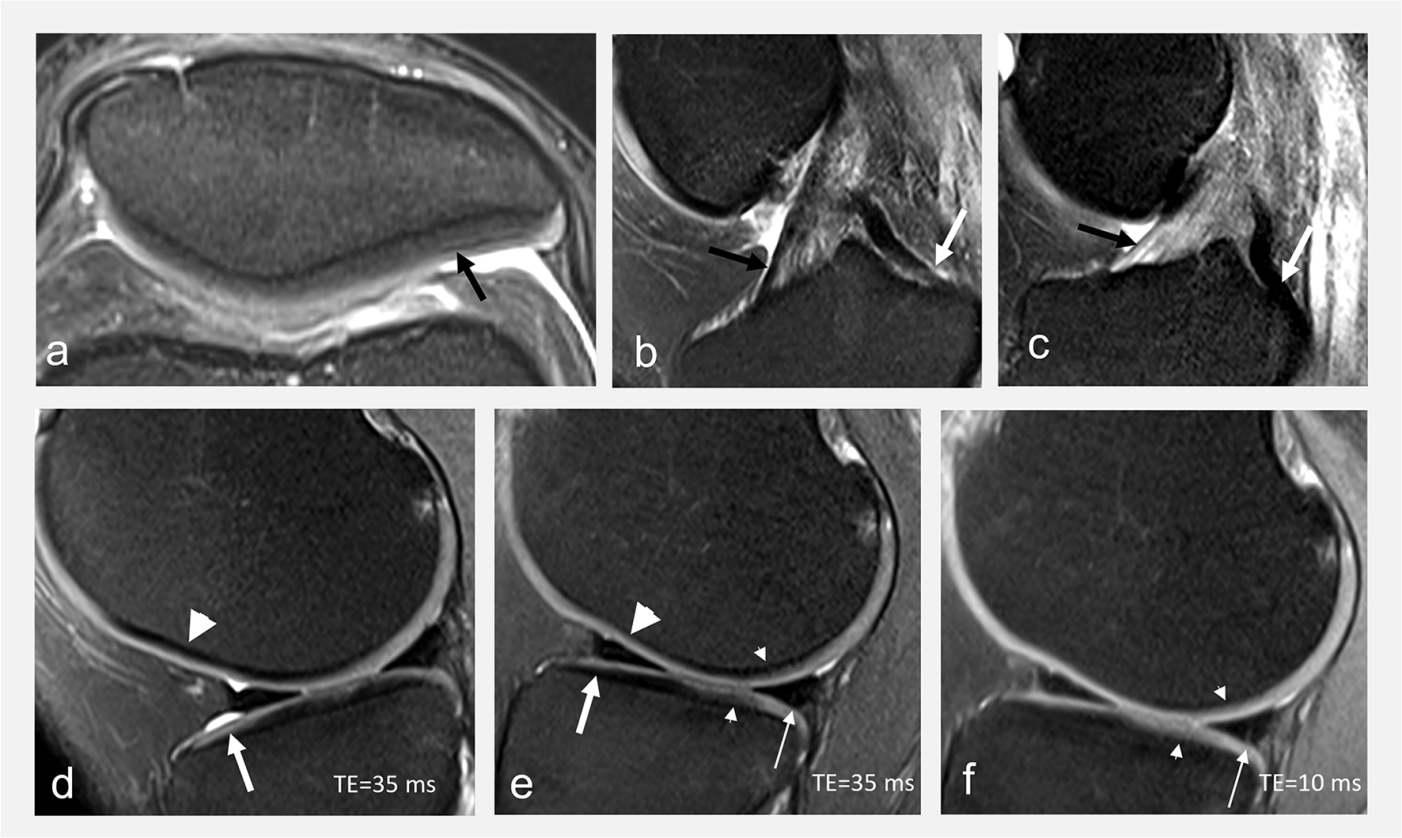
a Transverse FS IW MR image obtained on a 3T MRI unit showing truncation artifact (arrow in a) that gives a “false” laminar appearance to the patellar cartilage. Truncation artifacts typically appear as fine parallel lines immediately adjacent to high-contrast interfaces. Sagittal FS IW images in b flexion and c extension in a 36-year-old asymptomatic volunteer showing a variable signal intensity of the anterior (black arrows in b and c) and posterior (white arrows in b and c) cruciate ligaments related to the magic angle effect. Sagittal FS IW images in d flexion and e extension, and f sagittal PD fat-suppressed images in extension in the same volunteer obtained on a 3T MRI unit: the signal intensity of the cartilage (arrows) and the chondro-osseous junction (arrowheads) varies according to the orientation within the main magnetic field due to the magic angle effect. The signal intensity of cartilage (small arrows in e and f) also vary according to the TE. Note the fine meniscal high signal intensity line (f) that does not univocally reach the surface, seen on one image, and not visible on IW FS images (e), interpreted as no tear
